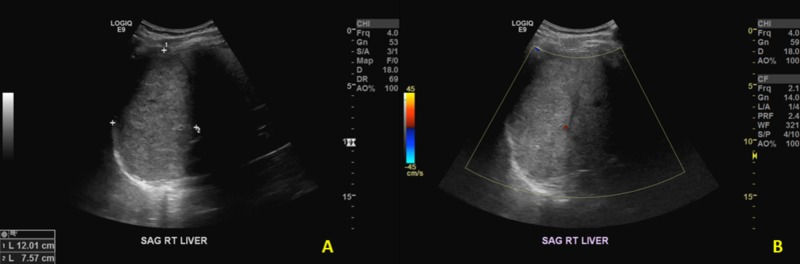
Liver ultrasound, sagittal view A. Sagittal ultrasound image of the liver demonstrating a hyperechoic mass within the liver with increased posterior through transmission. B. Doppler evaluation of this mass reveals no significant internal flow
